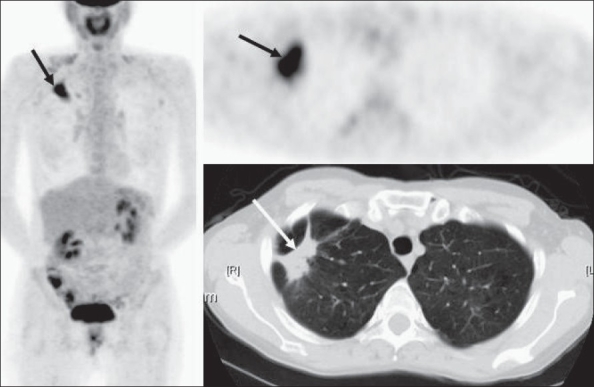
Axial PET (top right) and CT scan (bottom right) images at the level of the upper lungs, with an MIP image (on left), from a PET study of a 46-year-old lady with a suspicious right upper lung mass and with a significant smoking history. Intense FDG uptake corresponding to the mass (arrows) indicates a malignant etiology, unless proved otherwise; however, a resection biopsy showed only inflammation and necrosis
